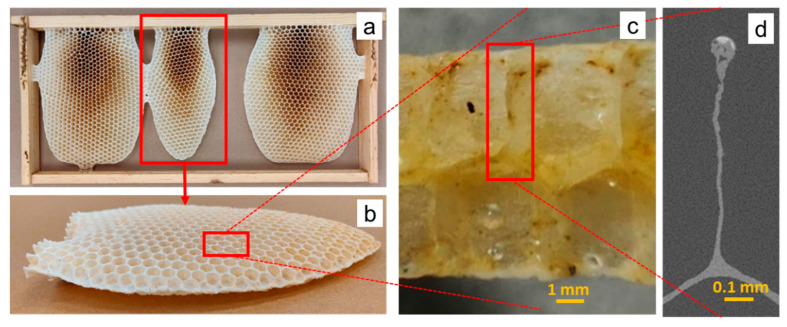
Coping in the walls of a bee’s natural honeycomb: (a) honeycomb made by *A. mellifera* in an open comb frame without any backing, (b) freshly built comb broken off, (c) laser-cut piece of comb, viewed side on, and (d) X-ray tomography slice showing that the cell wall terminates with clearly evident coping.
